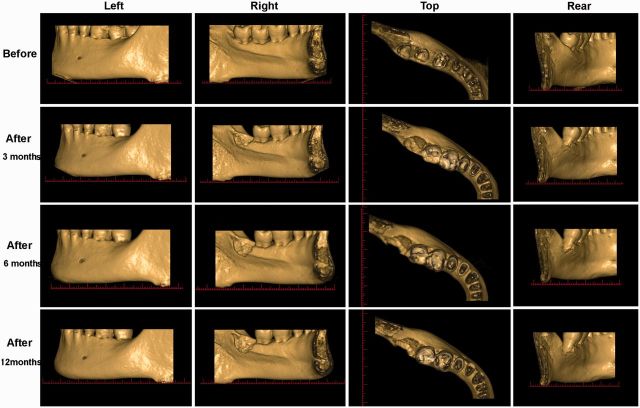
Three-dimensional CBCT reconstructions of experimental site from left, right, top and rear perspectives at different time points.
